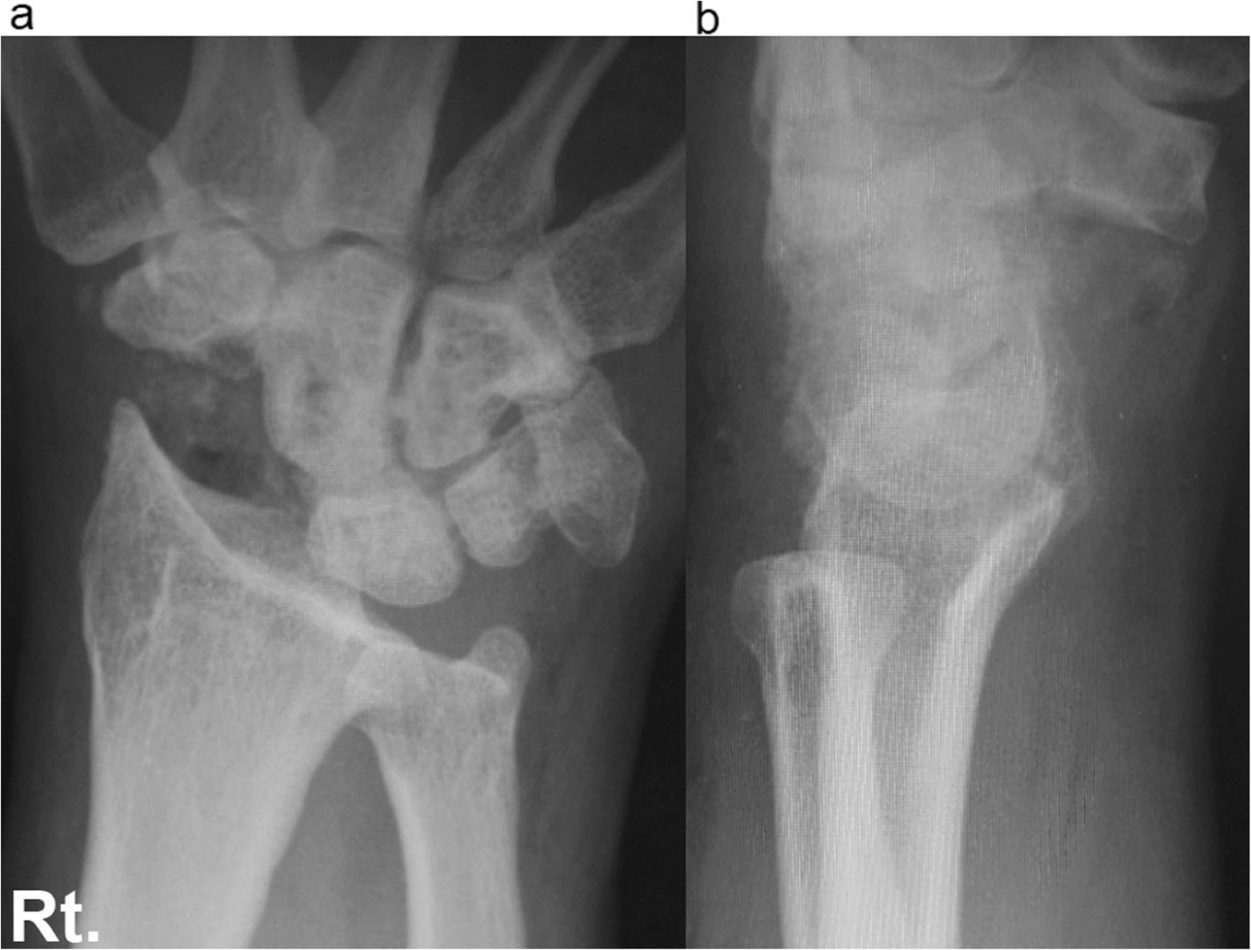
Postoperative X-rays of a 56-year-old male at the one-year follow up
